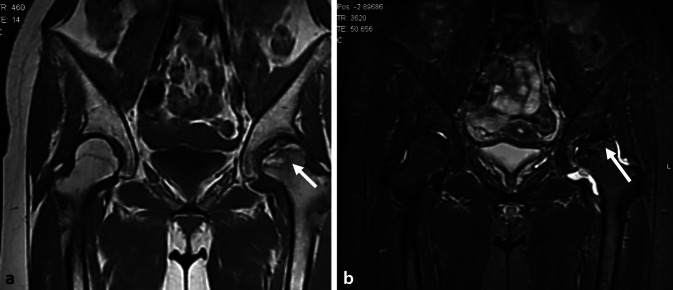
Chronic avascular necrosis (AVN) of the left femoral head. Coronal T1-weighted (a) and fluid-sensitive sequence (b) frontal views. There is a serpentine line representing the border of the AVN (*arrows*). There is fatty replacement of the necrotized part. The articular surface has collapsed. Osteoarthritis with cartilage loss and joint synovitis are also seen
